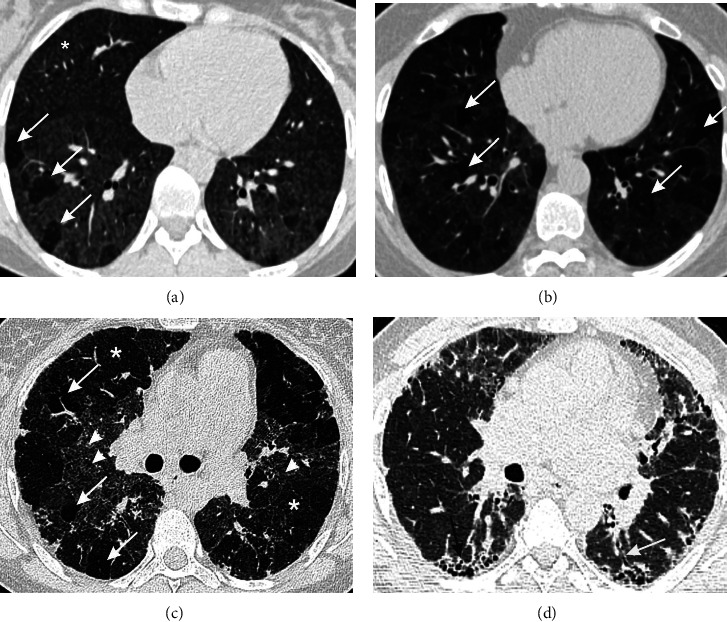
Imaging patterns in HP. (a) Axial section of chest high-resolution computer tomography (HRCT) showing ill-defined centrilobular nodules (white asterisk) and areas of air-trapping (arrows) suggesting small airway involvement. (b) Ground-glass opacities and mosaic attenuation (ground glass alternating with air-trapping (arrows)) suggestive of predominant interstitial infiltration. (c) The “three density sign” comprised of lung lobules with normal density (white asterisk), lobules with ground-glass attenuation (arrowheads), and lobules with decreased density due to air-trapping (arrows). (d) Fibrotic HP presented by areas of ground glass accompanied by traction bronchiectasis (black arrow) and honeycombing (white arrow).
